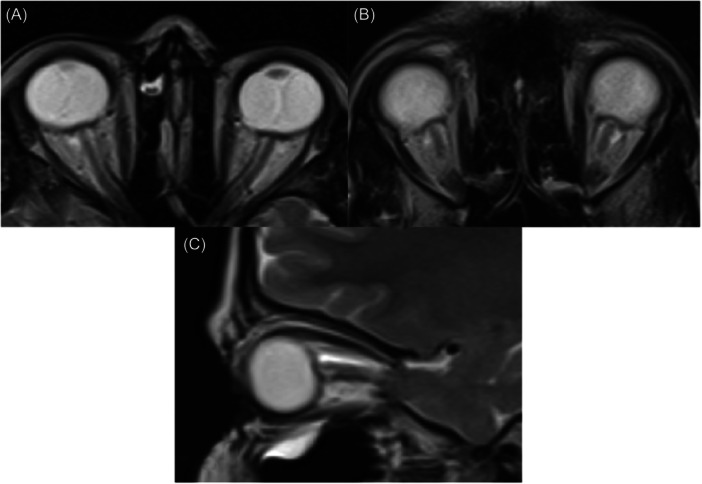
Posterior globe flattening. Axial (A and B) and sagittal (C) T2‐weighted MRI show disturbance of the normal convexity and straightening of the globe's outward curvature at its attachment to the optic nerve. MRI, magnetic resonance imaging.
